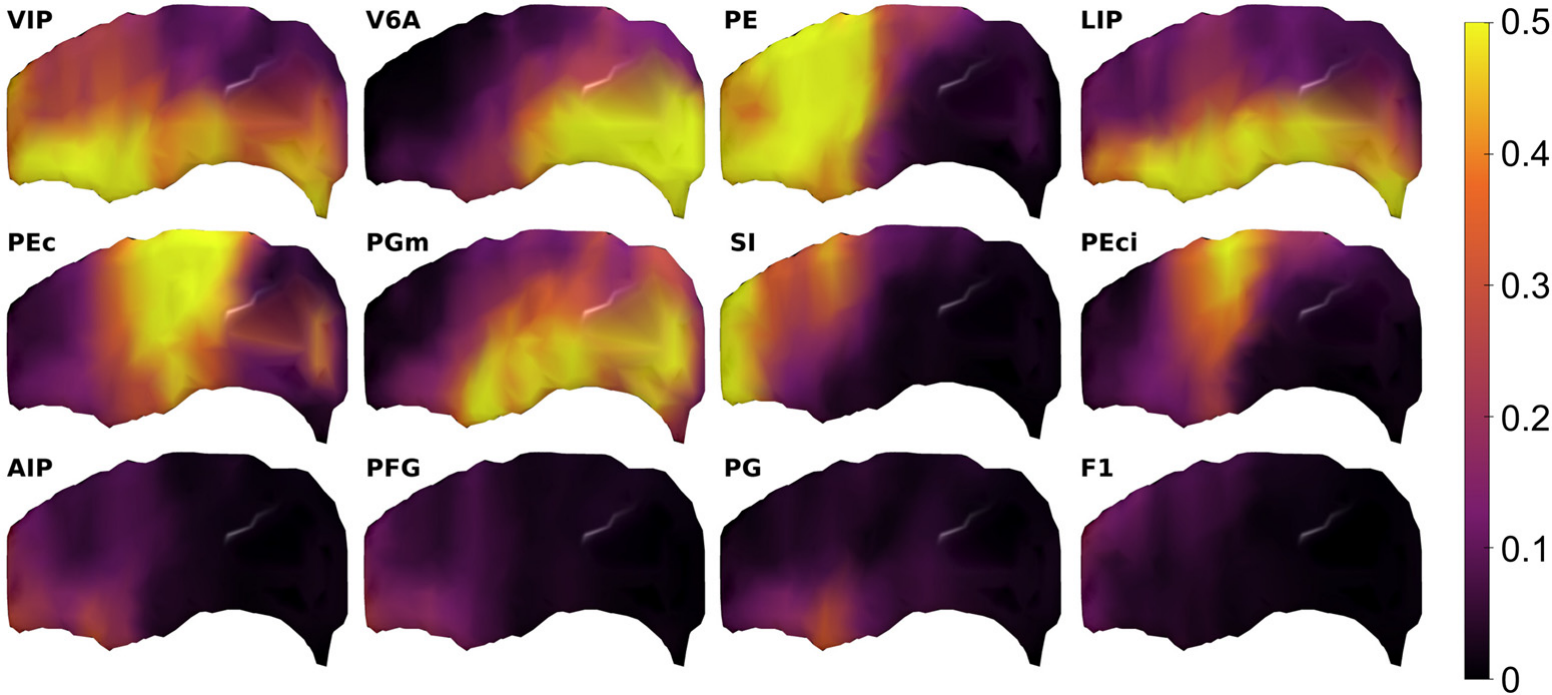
Spatial distribution of the IPS connectivity estimated from DW-MRI along 34 dorsal, middle, and ventral A-P sectors of the db-IPS, for the 12 cortical ROIs displaying the strongest estimated connectivity with the db-IPS (Fig. 11). The yellow and orange regions highlight the IPS locations with the strongest estimated connectivity for the corresponding cortical areas.
